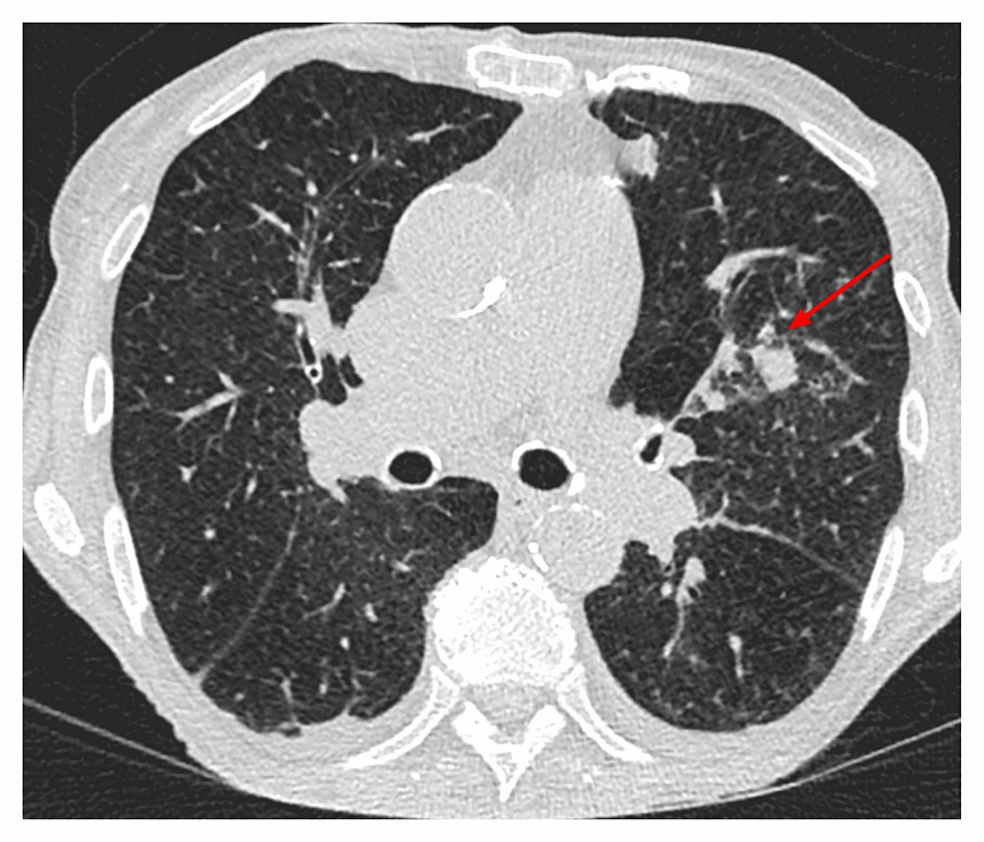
Computed tomography scan of the chest demonstrating left-sided pulmonary consolidations.
